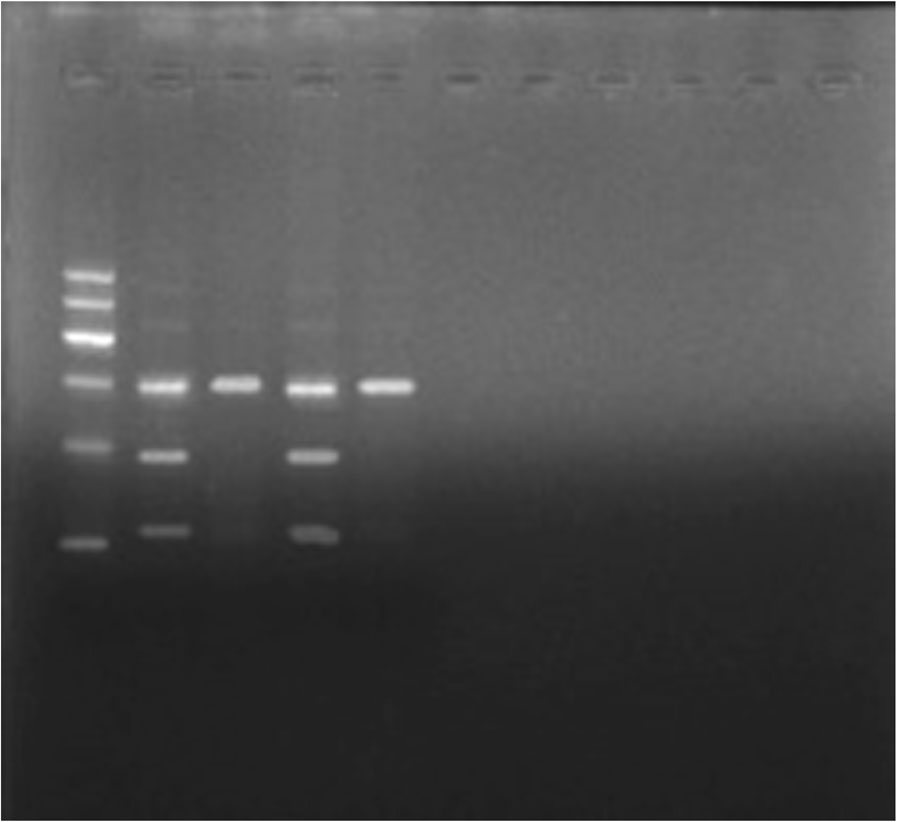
GR G678S. Lanes 2, 4: CC genotype; Lanes 1, 3: CT genotype; Lane M: 100 bp ladder
